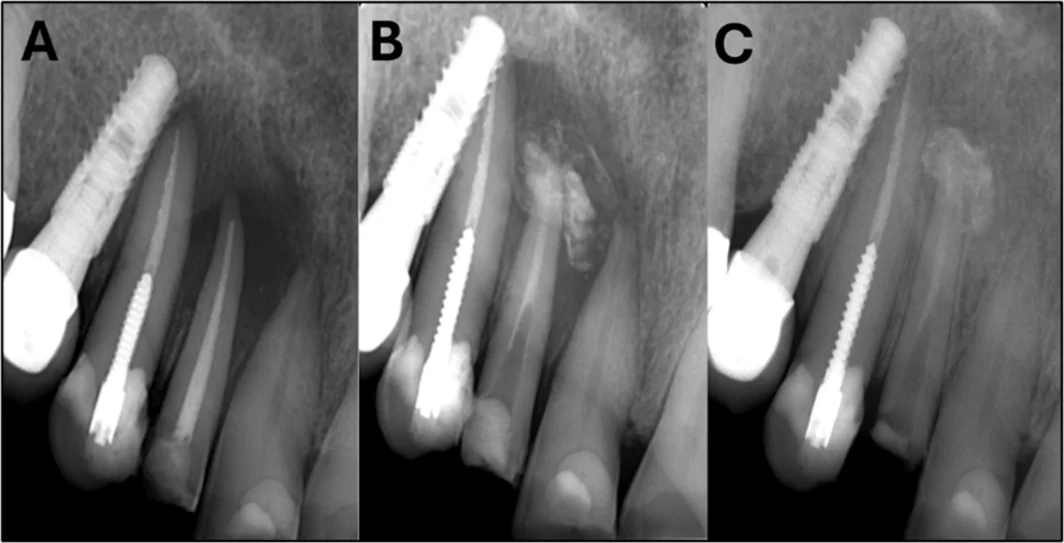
Case 3. (A) Preoperative periapical radiograph demonstrating a large periapical radiolucency associated with previously treated tooth #7. (B) Immediate postoperative radiograph following placement of a 5‐mm CC apical plug, with extrusion of CC beyond the root apex. (C) Two‐year follow‐up radiograph demonstrating that the previously observed periapical radiolucency was no longer evident.
